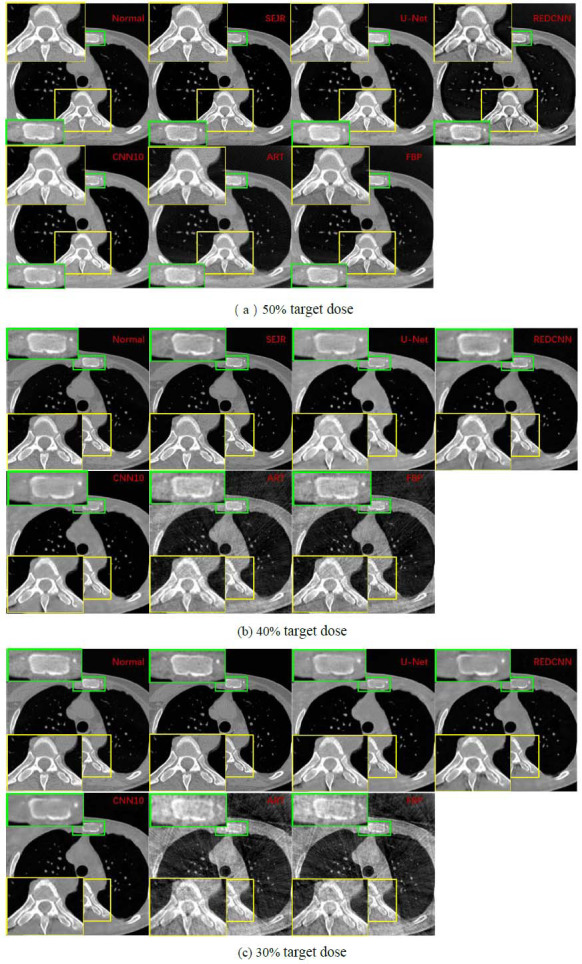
Two regions of interest (ROIs) from the visual differences of the different target dose CT image in the AAPM dataset reconstructed by SEJR and other methods were selected for detailed comparison.
